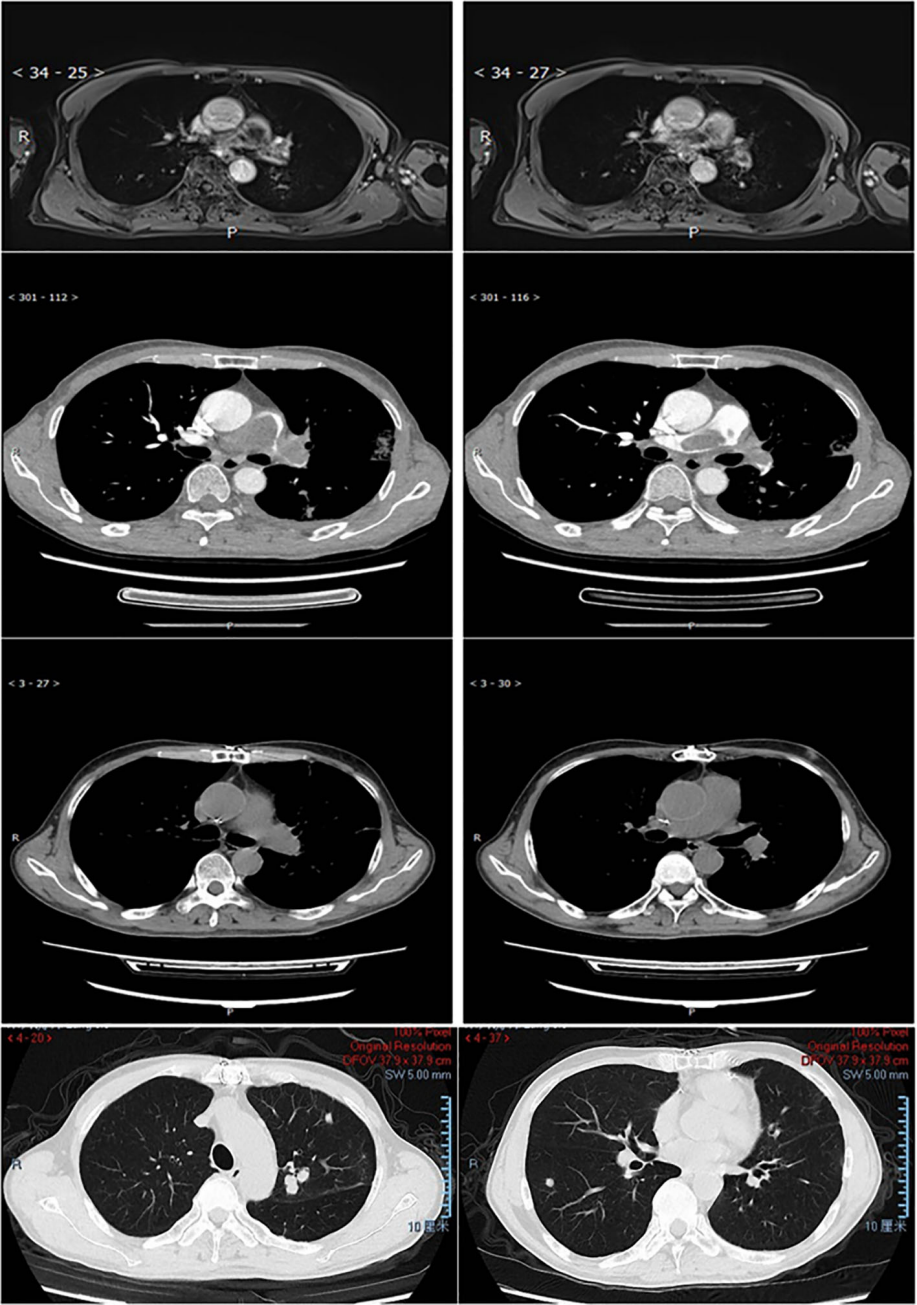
MRI/CT scan of the lung in patient P007. Row 1 (MRI) 2 (CTA), pulmonary artery intimal sarcoma involved PT, LPA, and RPA at diagnosis. Row 3-4, 12 months after PEA the patient relapsed with tumor involved left upper lobe, right lower lobe, and multiple little nodules in the peripheral lung.
